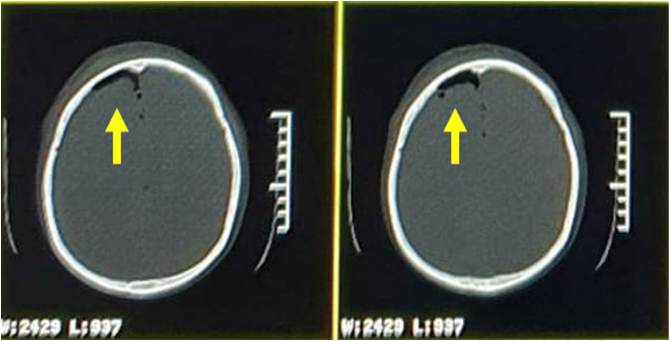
CT scan of bone window showing right frontal PNC.
